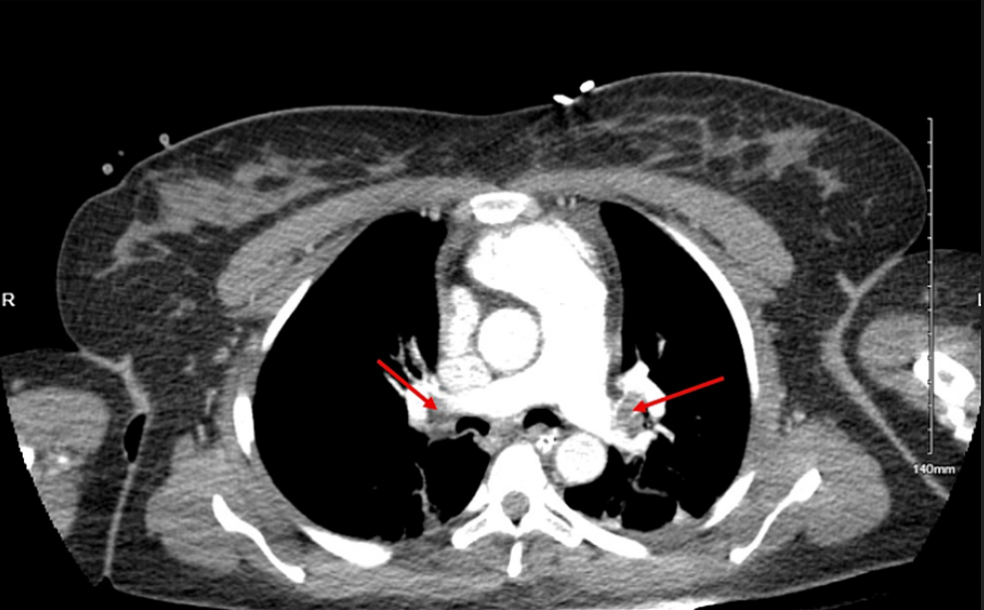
CT chest with contrast demonstrating bilateral embolisms (red arrows) with grossly dilated pulmonary artery
